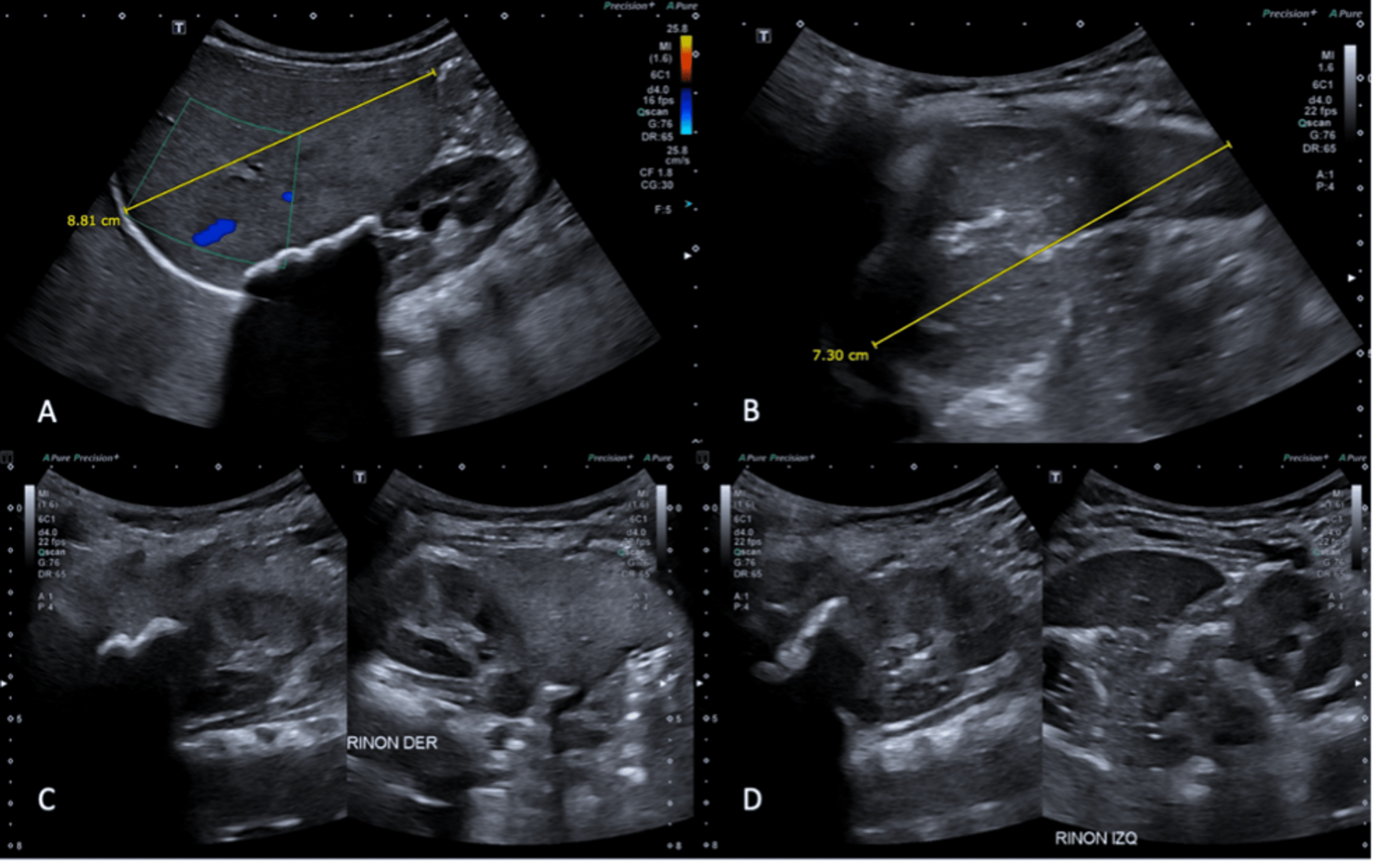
Selected images from a complete abdominal ultrasound examination. (A,B) Hepatosplenomegaly with diffuse increased echogenicity of the hepatic parenchyma, consistent with hepatic steatosis. (C,D) Diffuse enlargement of the adrenal glands with lobulated echogenic foci producing posterior acoustic shadowing, compatible with parenchymal calcifications
